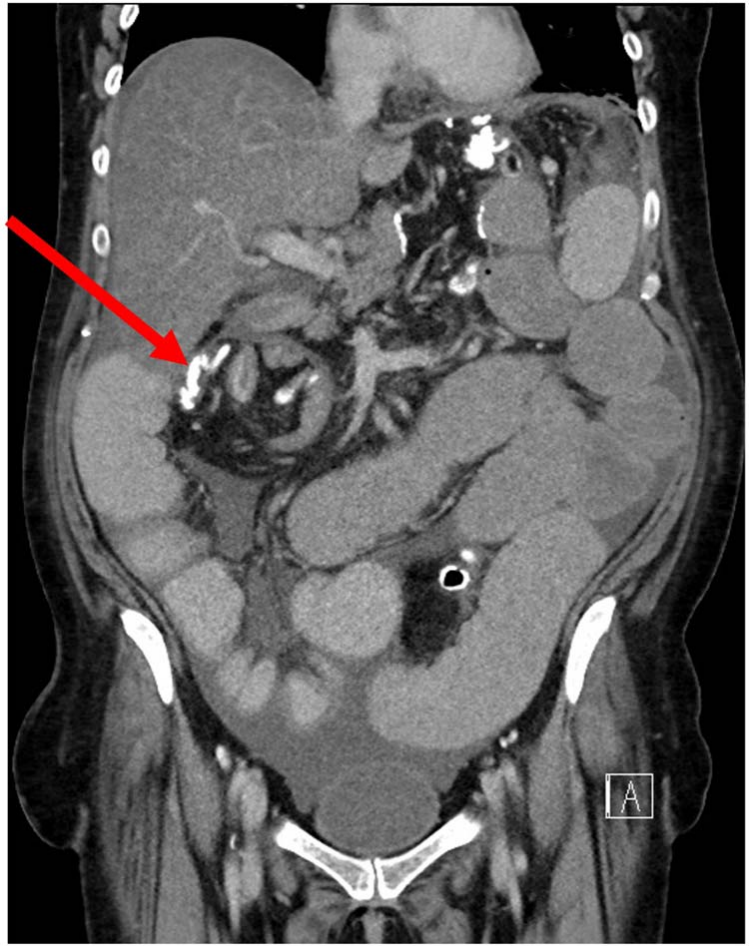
Coronal CT of SBO secondary to internal hernia.
